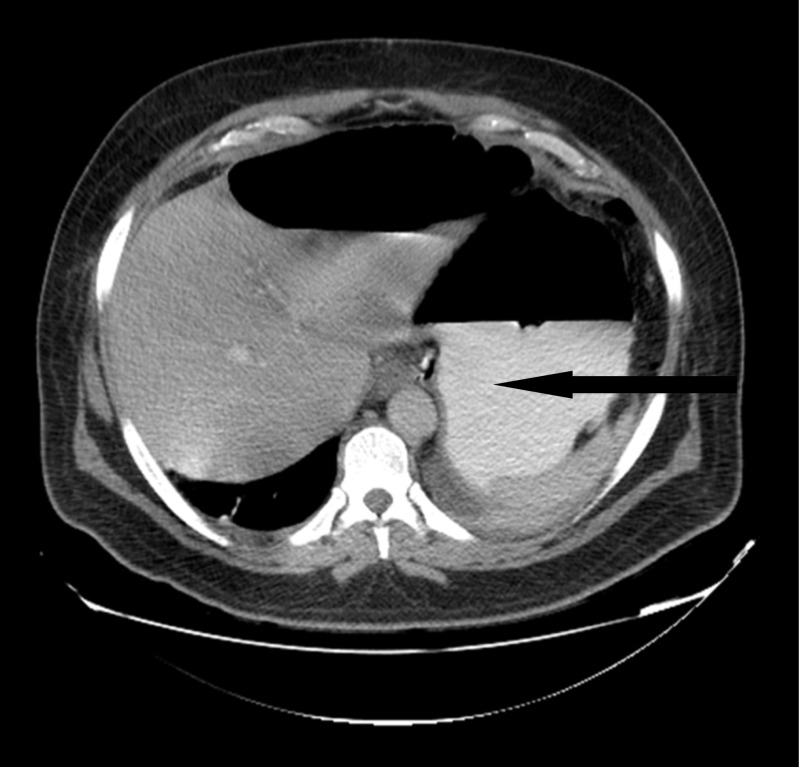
CT scan showed perigastric tube air-fluid collection
